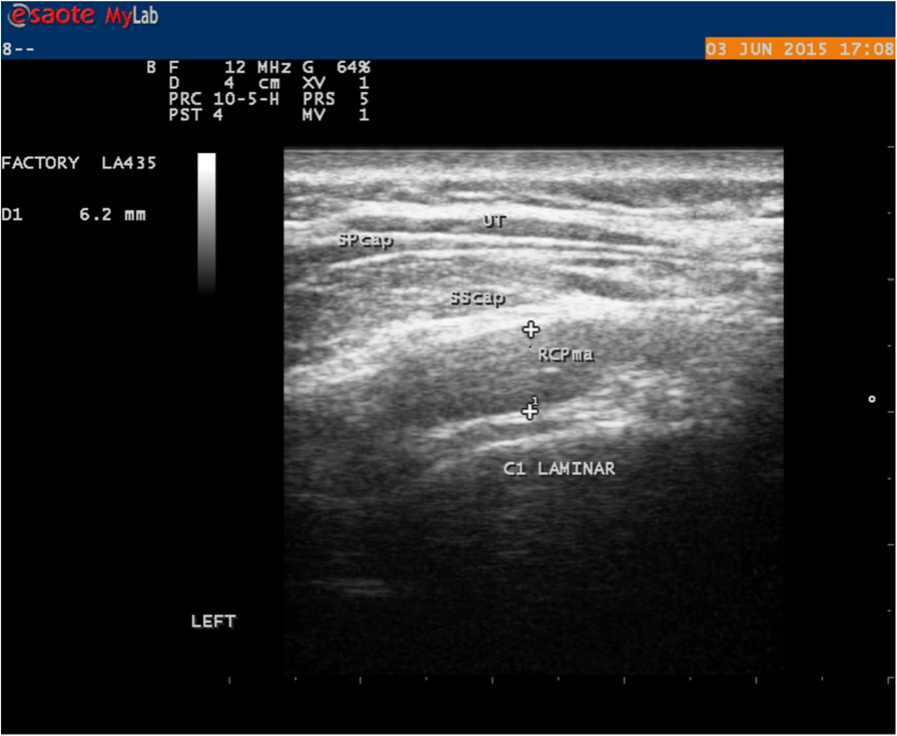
Ultrasound image of the Rcpm at rest in transverse view at C1. The caliper is placed around the midpoint above the C1 lamina betwen the inner and outer borders of the muscle where it was considered thickest by the raters
